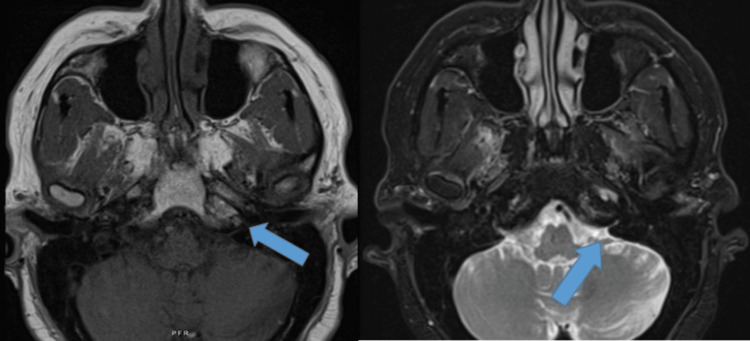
Coronal T1 and T2-weighted brain MRI showing a small focus of extra-axial restricted diffusion in the left cerebellopontine angle cistern with associated enhancement and increased enhancement along the adjacent tentorium.
